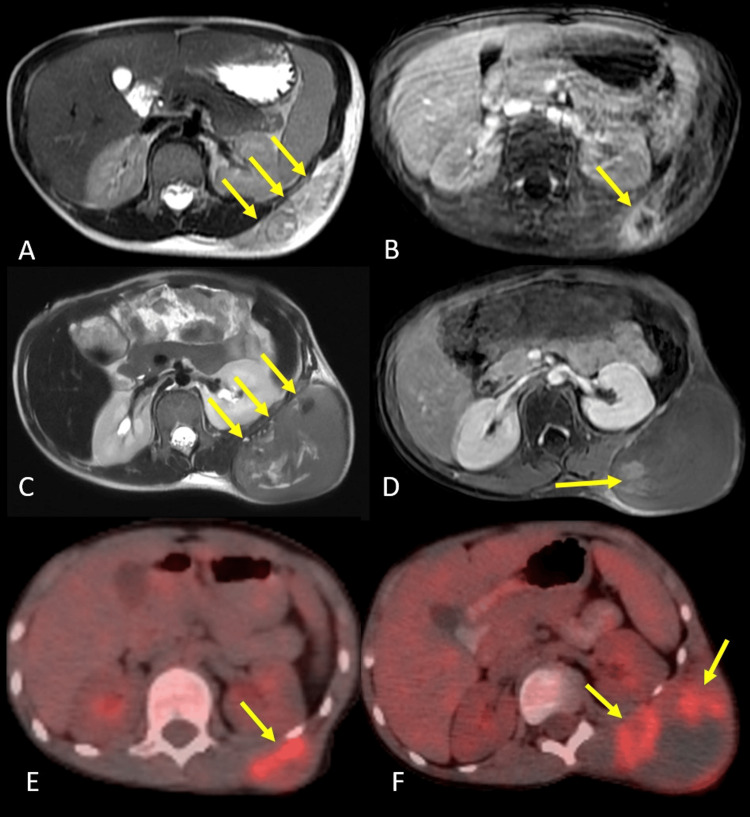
Baseline and follow-up imaging findings. At the time of diagnosis, (A) axial T2-weighted MR image shows a heterogeneous mass (arrows) in the subcutaneous fat tissue, and (B) axial postcontrast T1-weighted MR image shows a contrast-enhancing nodular component (arrow) within the mass. After induction chemotherapy, (C) axial T2-weighted MR image shows progression of the mass (arrows), and (D) axial postcontrast T1-weighted MR image shows a contrast-enhancing component (arrow) within it. Comparative PET-CT scans taken (E) at the time of diagnosis and (F) after induction chemotherapy, before RT, show an increase in mass size and FDG uptake (arrows). MR, magnetic resonance; PET-CT, positron emission tomography-computed tomography; FDG, fluorodeoxyglucose; RT, radiotherapy
